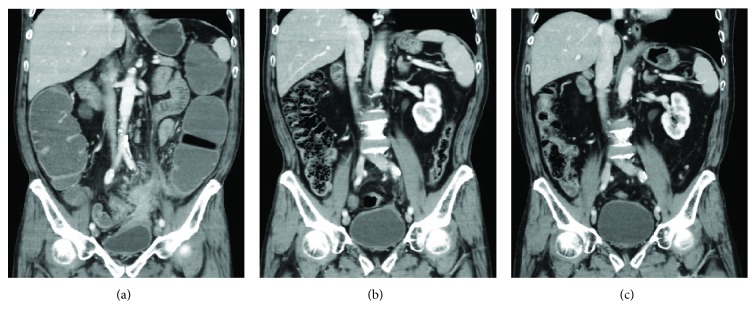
Coronal section of CT indicated the efficacy of neoadjuvant chemotherapy. Before chemotherapy (a). After 3 courses of CAPOX (b). After 5 courses, the tumor had disappeared (c).
